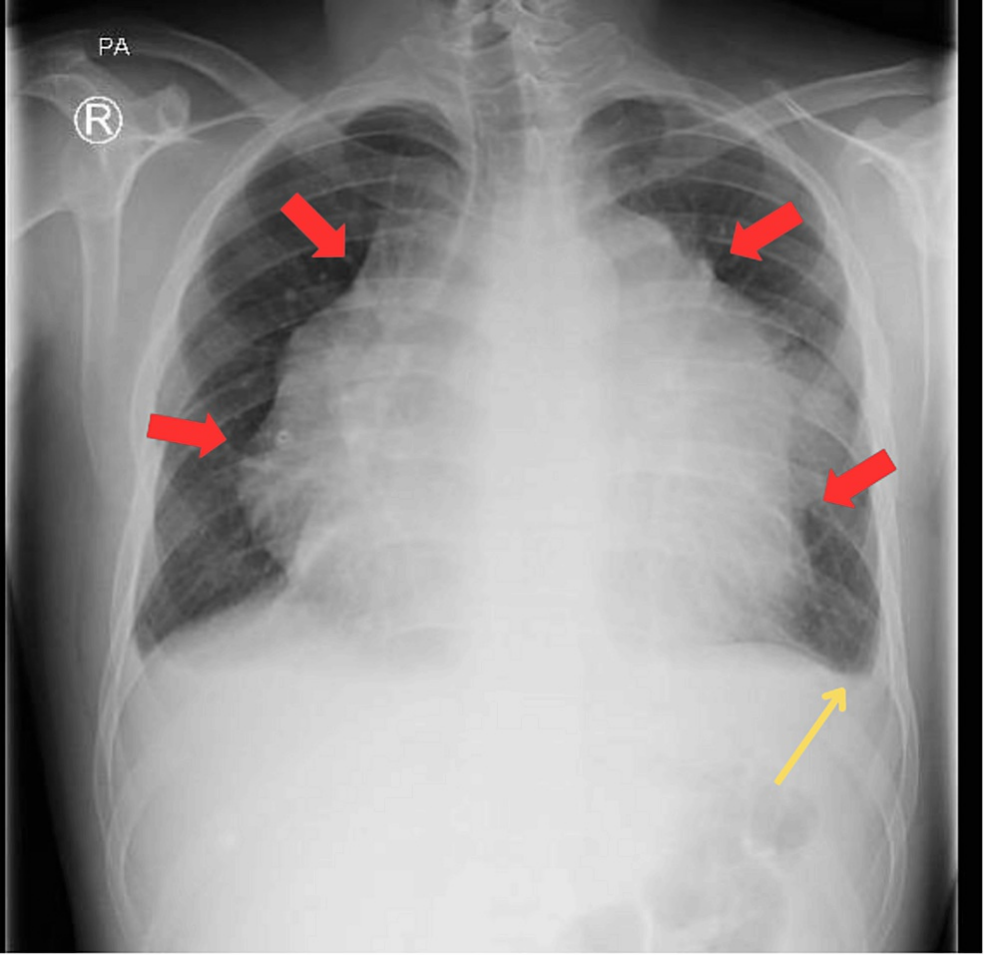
A posterior-anterior chest X-ray with red arrows surrounding the large mediastinal mass overlying the left lung field. Note the obliteration of the left costophrenic angle, which is pointed out by the yellow arrow.
